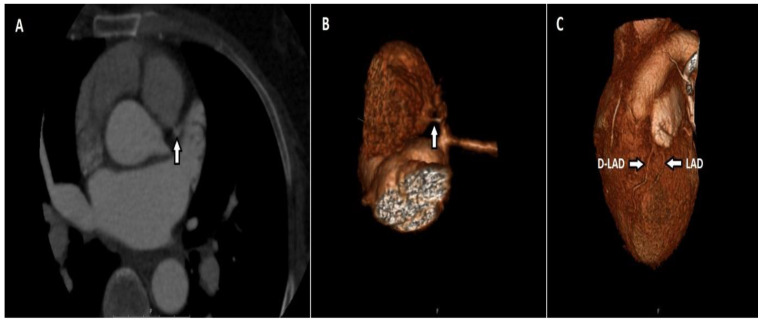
Axial CT maximum-intensity projection (MIP) (A) and volume-rendered (VR) (B) image shows a thin fistula line between the LAD and the main pulmonary artery. A duplicated LAD (D-LAD) with an LAD is seen in the same patient (C).
